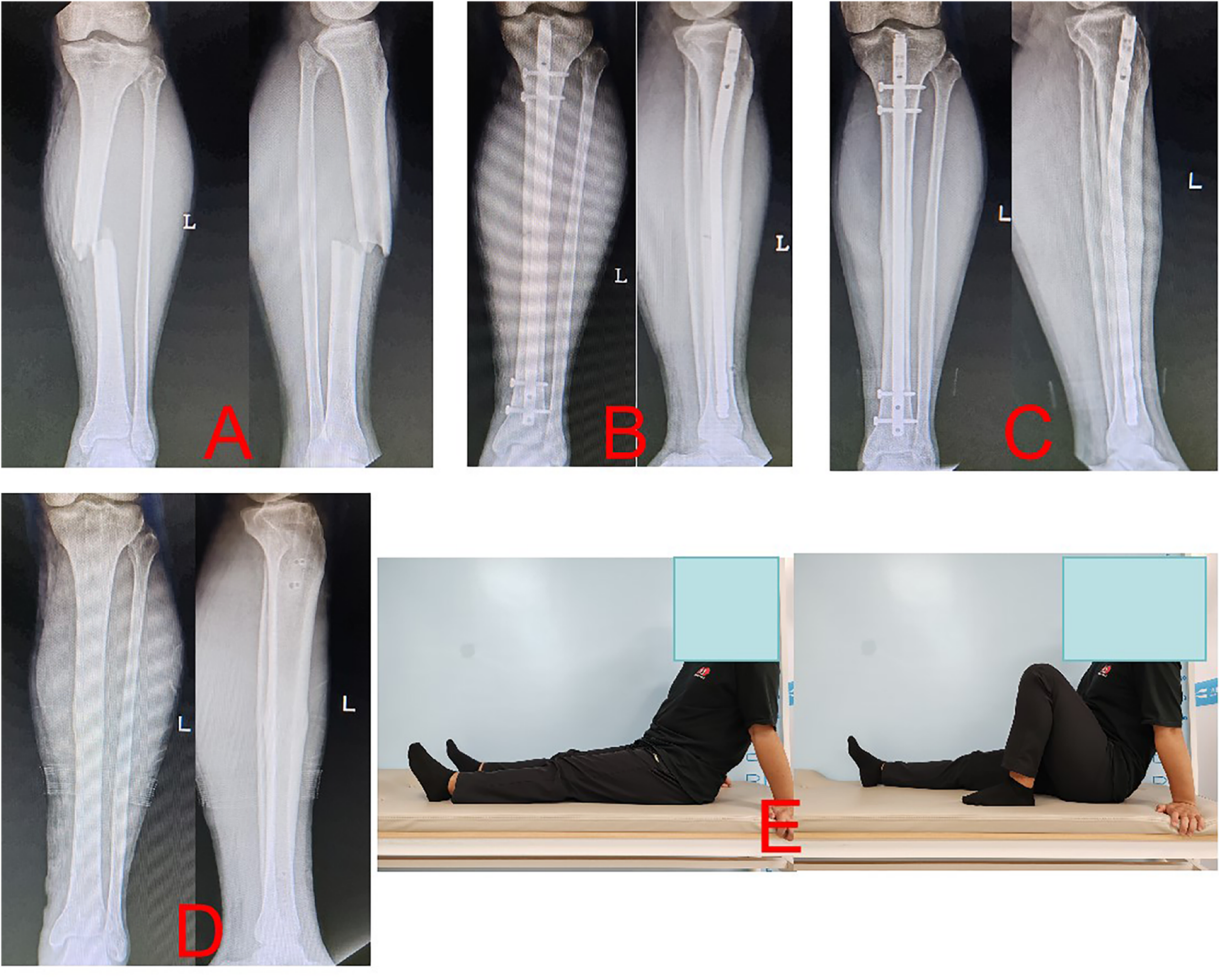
Radiographs of a case (a 33-year old man with the left tibia shaft fracture (AO- 42A3) of union after closed reduction using the LPE approach were presented. (A) Preoperative anteroposterior (AP) and lateral views. (B) AP and lateral views postoperatively. (C) AP and lateral views 13 months postoperatively. (D) AP and lateral views after IMN was removed. (E) Functional recovery at 13 months postoperatively.
